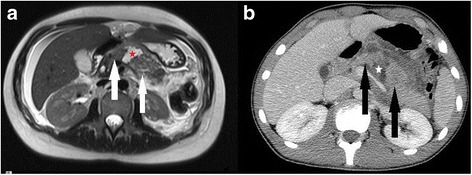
T2-weighted magnetic resonance imaging (a) of patient 1 and computed tomography (b) of patient 2 depicting complete rupture of the pancreas (*white* and *black arrows*). Fluid collection between the ruptured pancreas (*star*)
